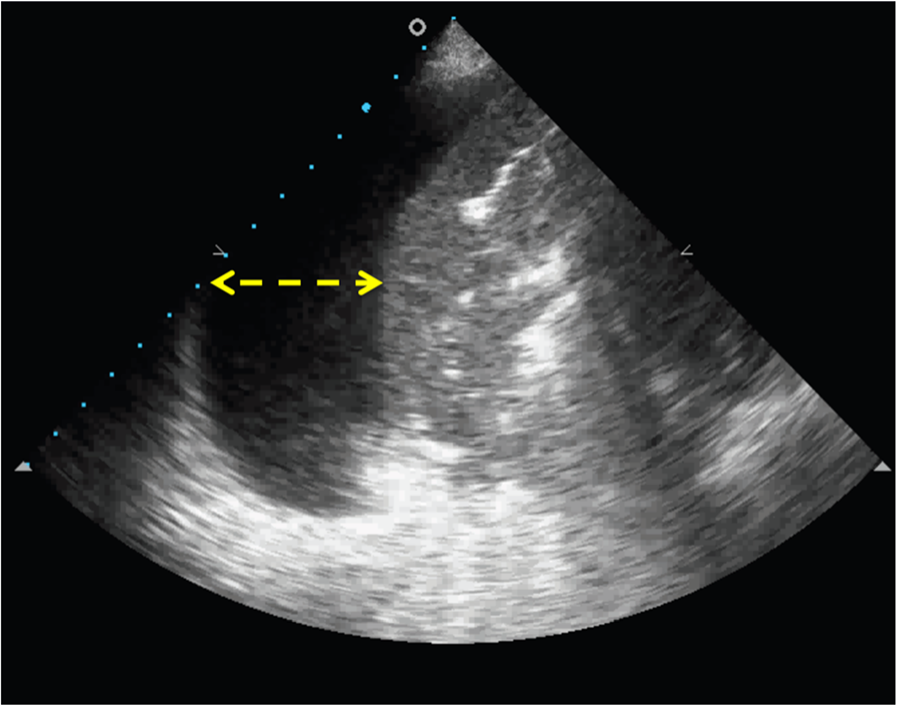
Ultrasound technique to measure the pleural effusion (in centimeters), from chest wall to pulmonary parenchyma (*dashed line*)
